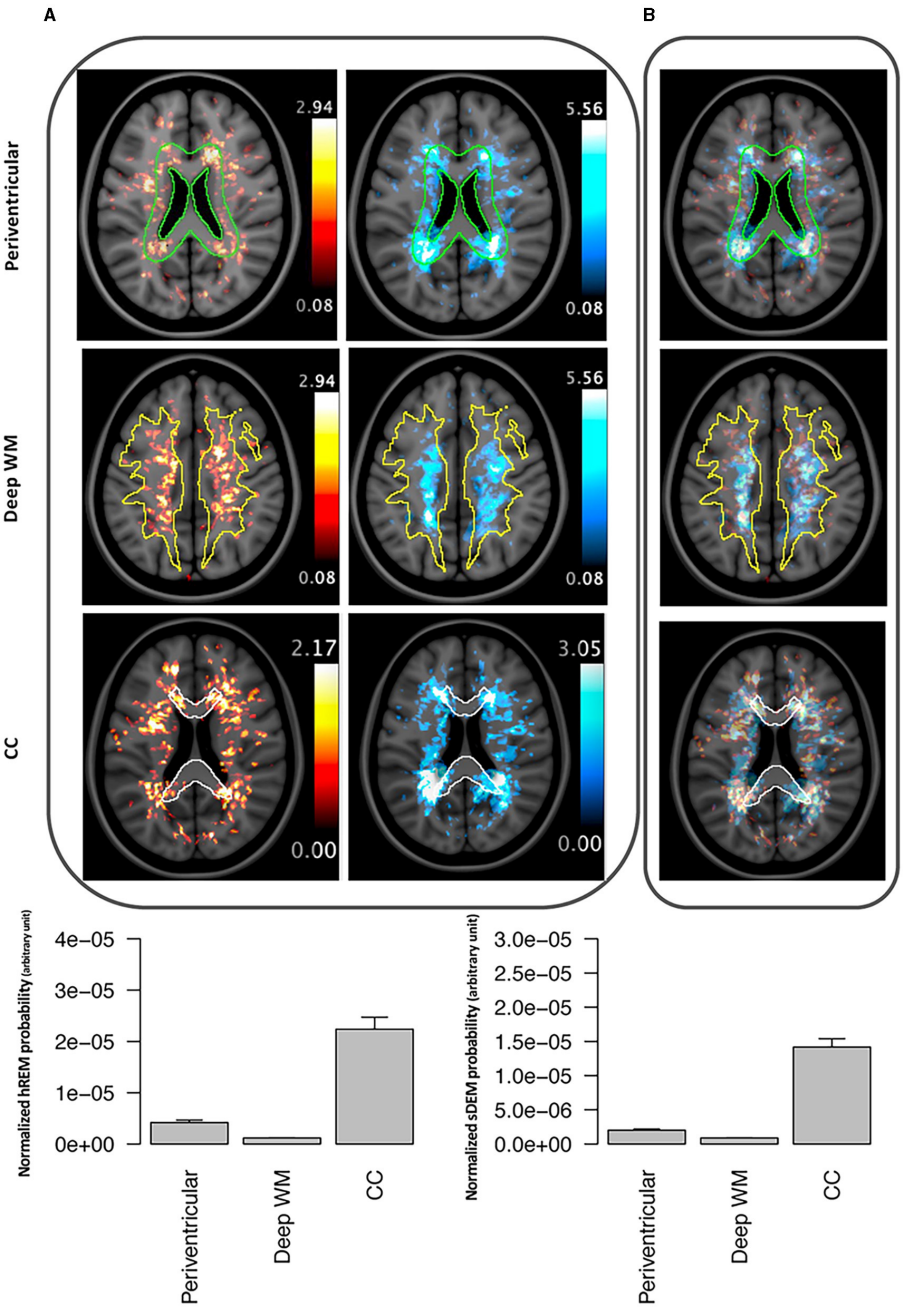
Example lesion distribution maps and area-wise quantification across all RRMS participants. Top panel shows the distribution of severely demyelinated (red) and highly remyelinated (blue) lesions separately (A) and together (B) at 3 commonly recognized brain areas in MS: periventricular, deep white matter (WM), and corpus callosum (CC). All images represent corresponding MRI slices from the co-registered MNI template. The outlines wherein represent the area masks generated from the template. Bottom panel shows the mean (standard error) probability of each lesion type within each of the 3 defined brain areas. Individual probabilities are normalized by the volume of the corresponding anatomical area. sDEM, severely demyelinated lesions; hREM, highly remyelinated lesions.
